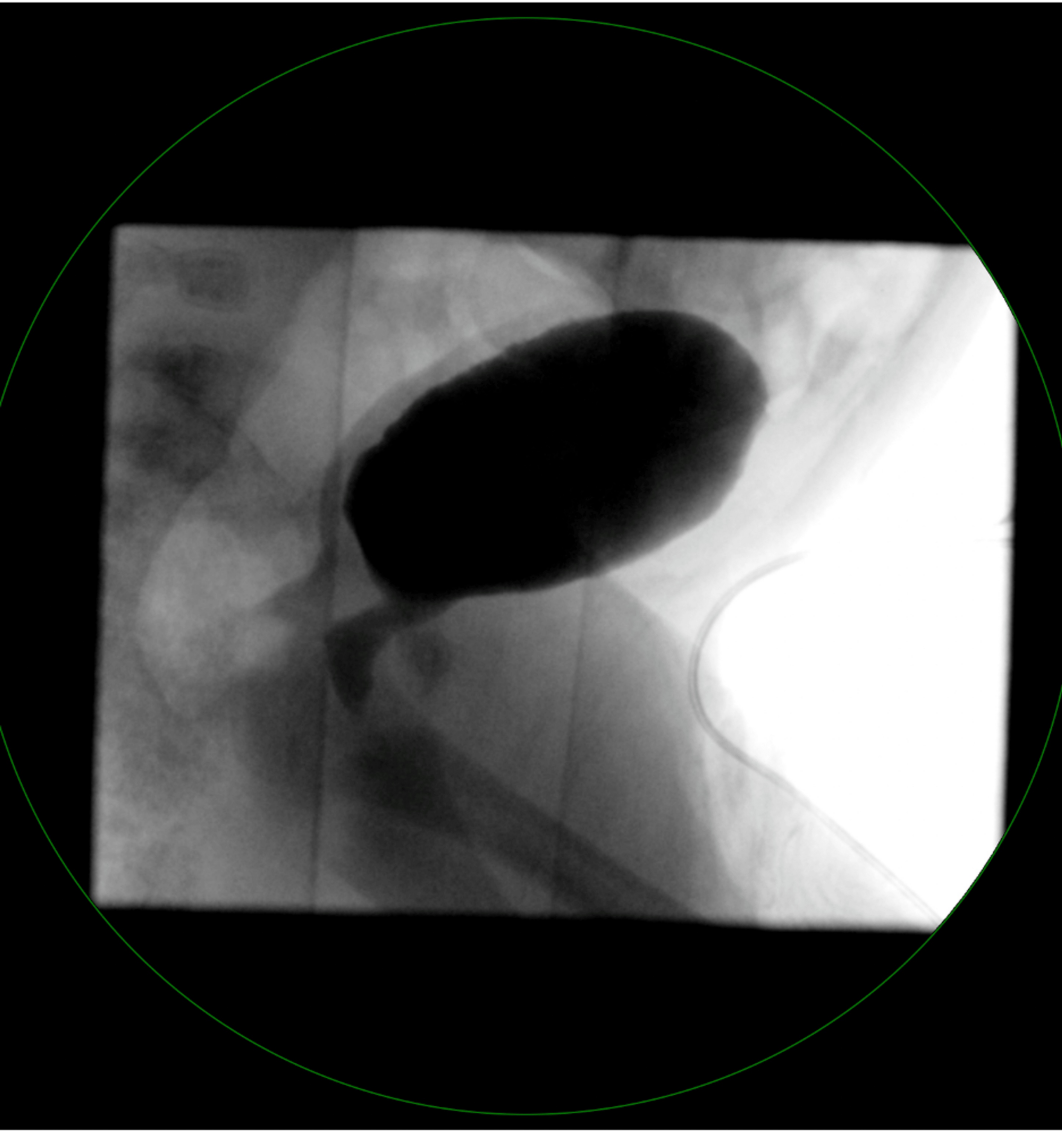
X-ray Urethrocystography Voiding Bladder urethrocystography appeared unremarkable, with no filling defects, and no vesicoureteral reflux
